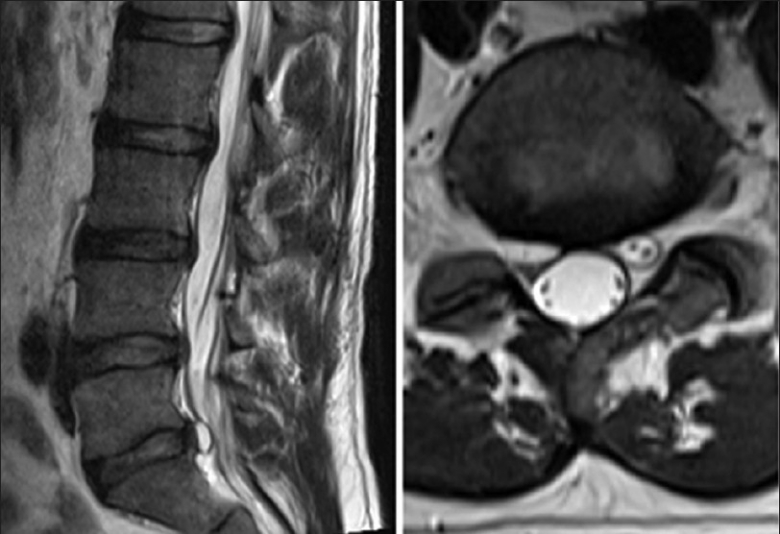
T2-weighted magnetic resonance image of a patient with right-sided S1 radiculopathy. Sagittal (left) and transaxial (right) views at the level of the L5-S1 disc demonstrate a T2 hyperintense cystic structure (arrow) extending into the right ventrolateral extradural space
